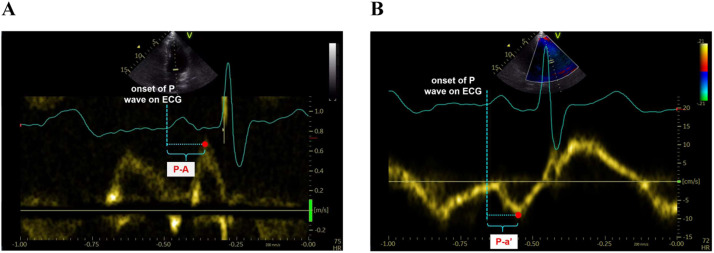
Left atrial electromechanical coupling measurement by pulse Doppler (A), and tissue Doppler imaging (B). P-A, The interval between the onset of the P wave and the peak transmitral flow A-wave; P-a’, The interval between the onset of the P wave and the peak septal/lateral mitral annulus a’-wave.
